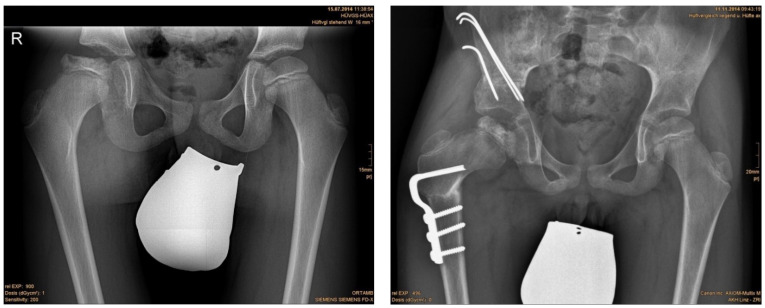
(left) Anteroposterior radiograph of a nine-year-old male with LCPD; (right): result three months postoperatively.
